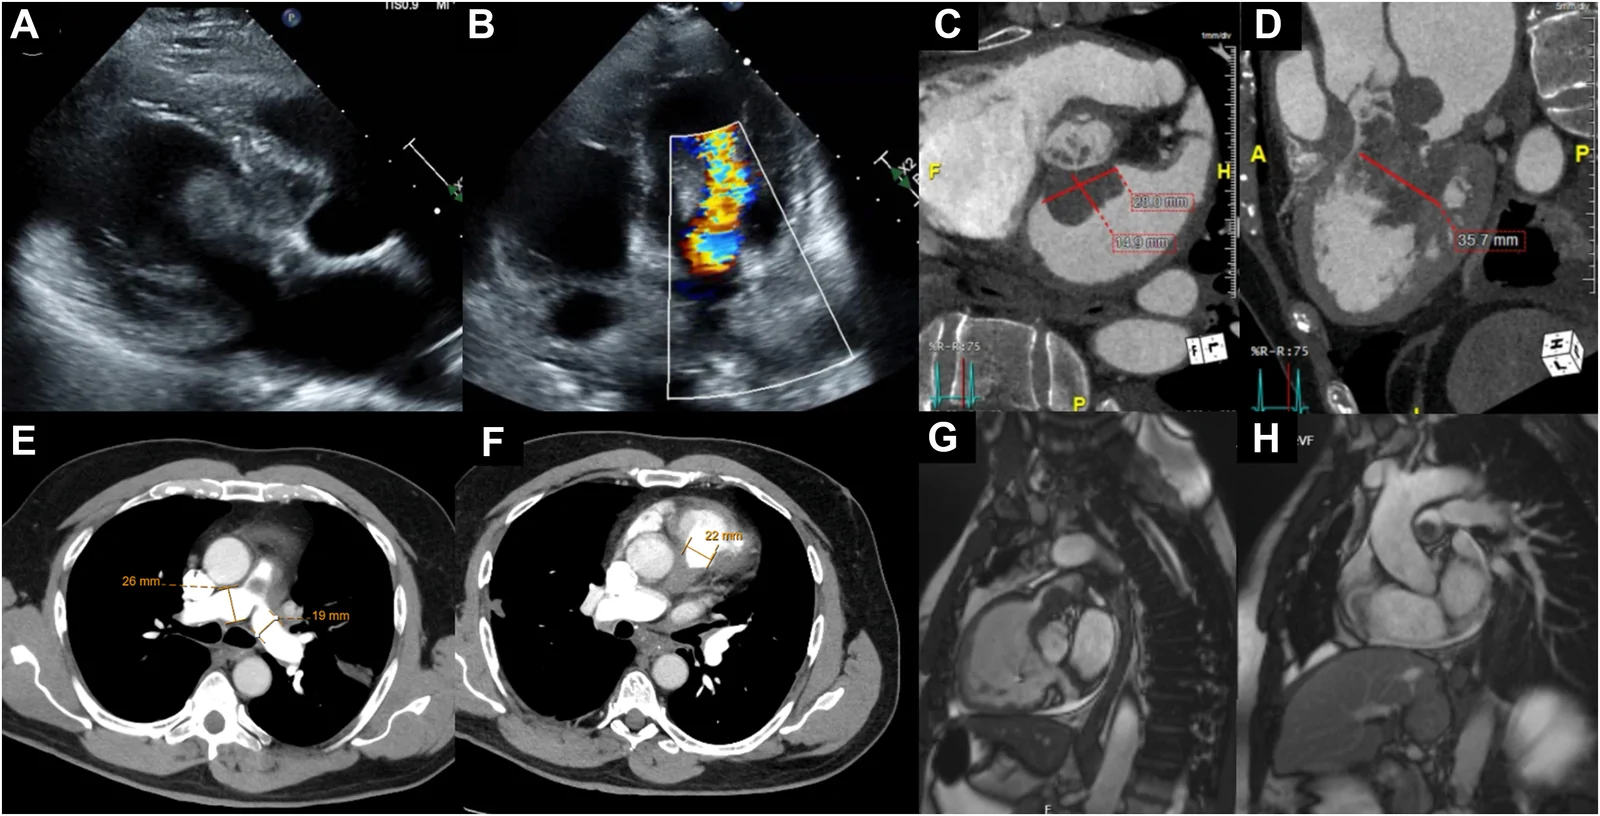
Preoperative imaging. (A-D) Patient 1. (A) Transthoracic echocardiogram demonstrating a large mass at the anterior mitral leaflet that extended into the left ventricular outflow tract. (B) Prolapse of the mass across the aortic valve during systole with severe functional mitral stenosis. (C, D) Computed tomography revealing a 36 mm × 34 mm × 18 mm heterogenous, multilobulated mass attached to the aortomitral curtain and posteromedial papillary muscle and resulting in nearly complete left ventricular outflow tract obstruction. (E-H) Patient 2. (E, F) Computed tomography demonstrating a 2.5 cm × 1.9 cm soft tissue mass along the right ventricular outflow tract and resulting in severe stenosis. (G, H) Magnetic resonance imaging showing a 17 mm × 22 mm heterogenous mass in the main pulmonary artery and extending into the proximal right pulmonary artery with obstruction.
